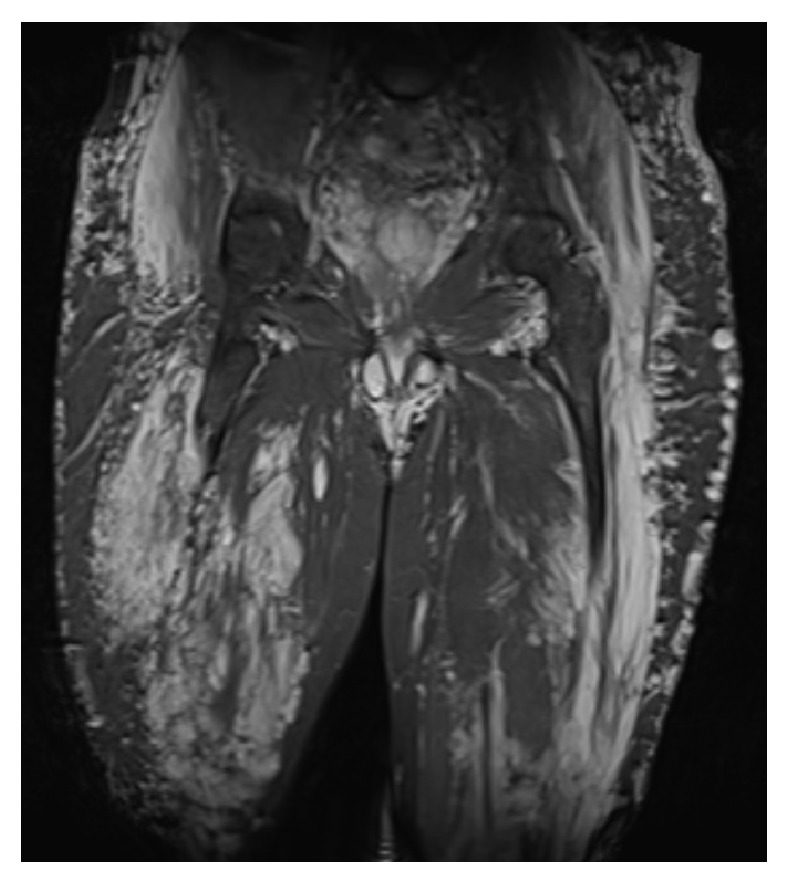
Coronal MRI STIR sequence of the anterior thigh.
